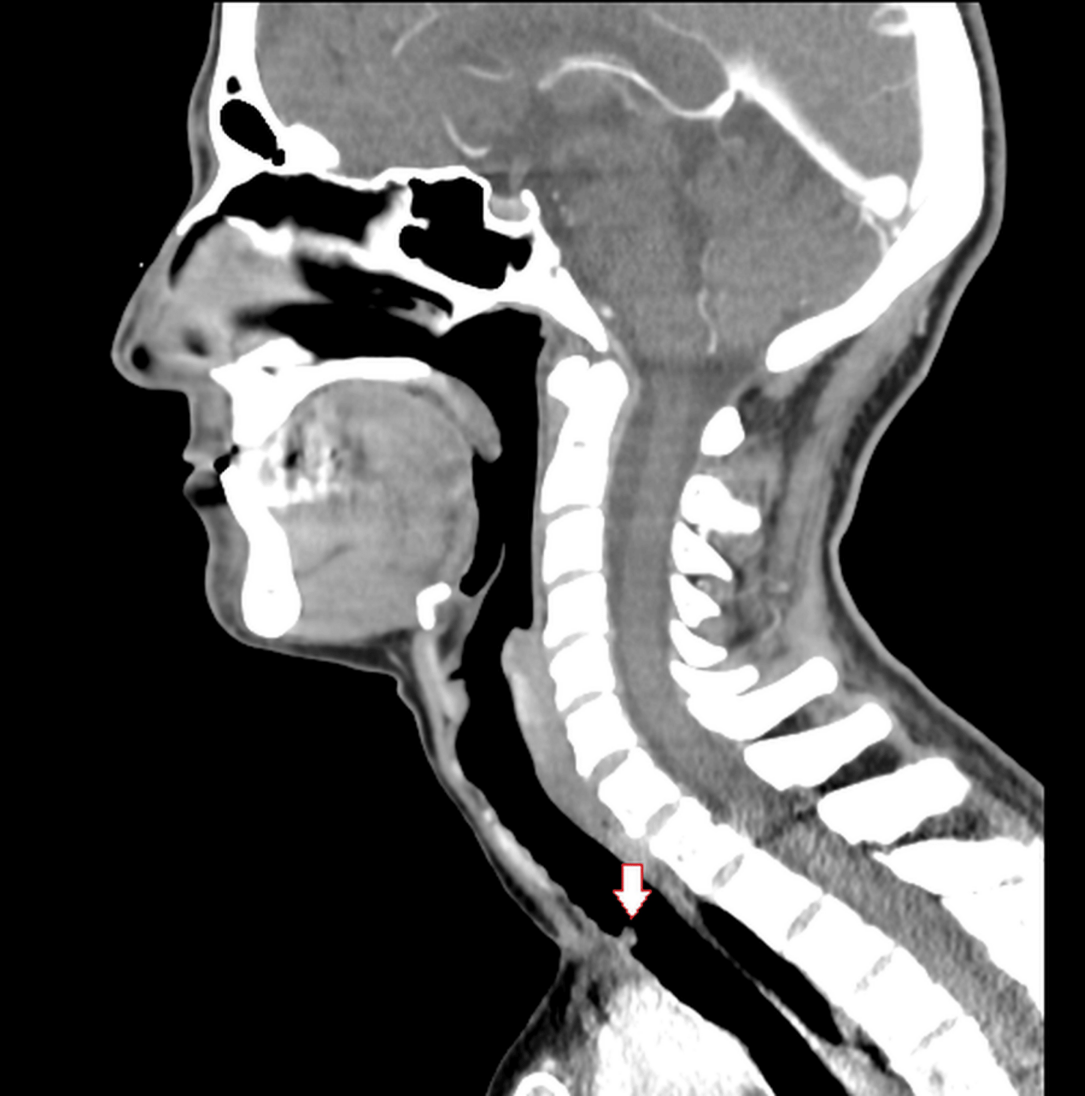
Sagittal computed tomography (CT) image showing an exophytic lesion within the tracheal lumen (arrow) located 7 cm above the tracheal carina
